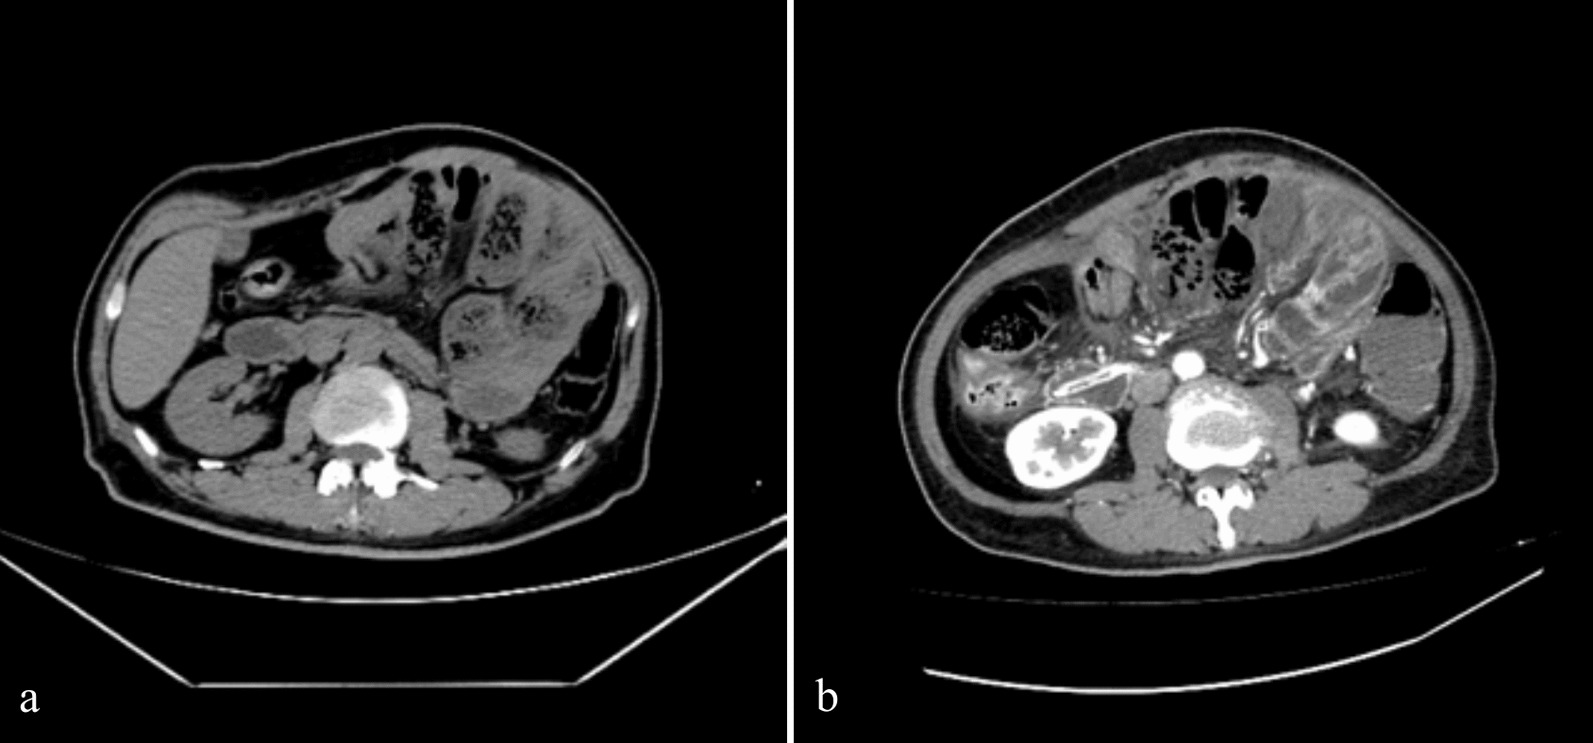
Computed tomography (CT) versus contrast-enhanced computed tomography. a Small intestine irregularly distributed and gathered in the left middle quadrant of abdomen indicating possible intraabdominal hernia; b contrast-enhanced CT scan obtained through the middle abdomen showing a conglomerate of multiple twisting and deformation intestinal loops surrounded by a enhancing thick sac-like structure
